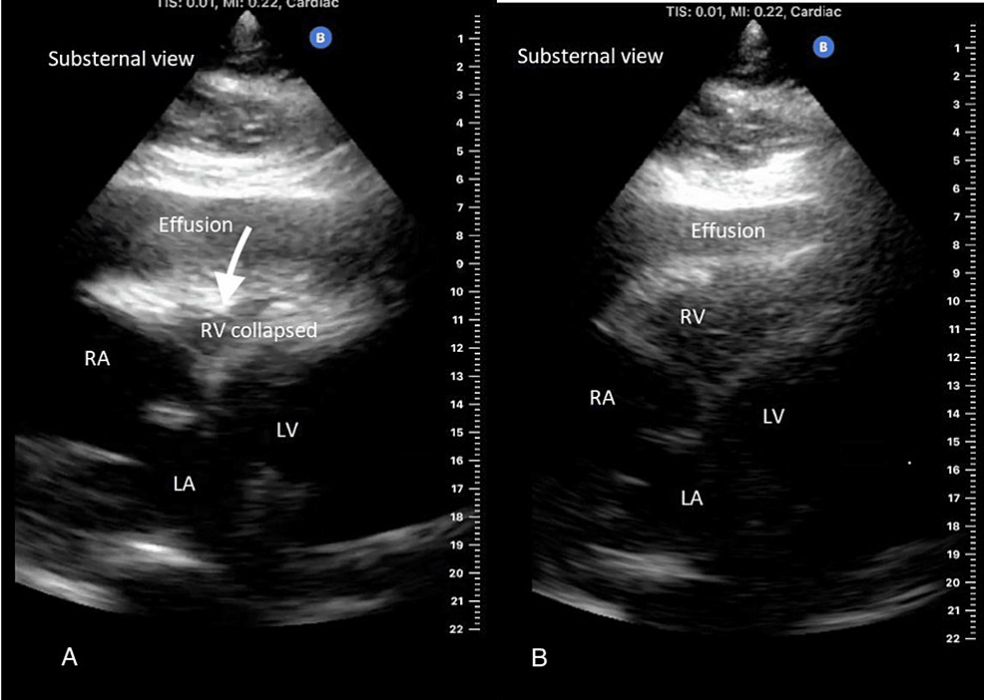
Bedside Echocardiography Bedside echocardiography using  Butterfly iQ+ - Handheld Ultrasound showing collapsing right ventricle during diastole (A) as compared to distended right ventricle during systole in (B). Abbreviations: RV- right ventricle, RA- right atrium, LV, left ventricle, LA- left atrium.
